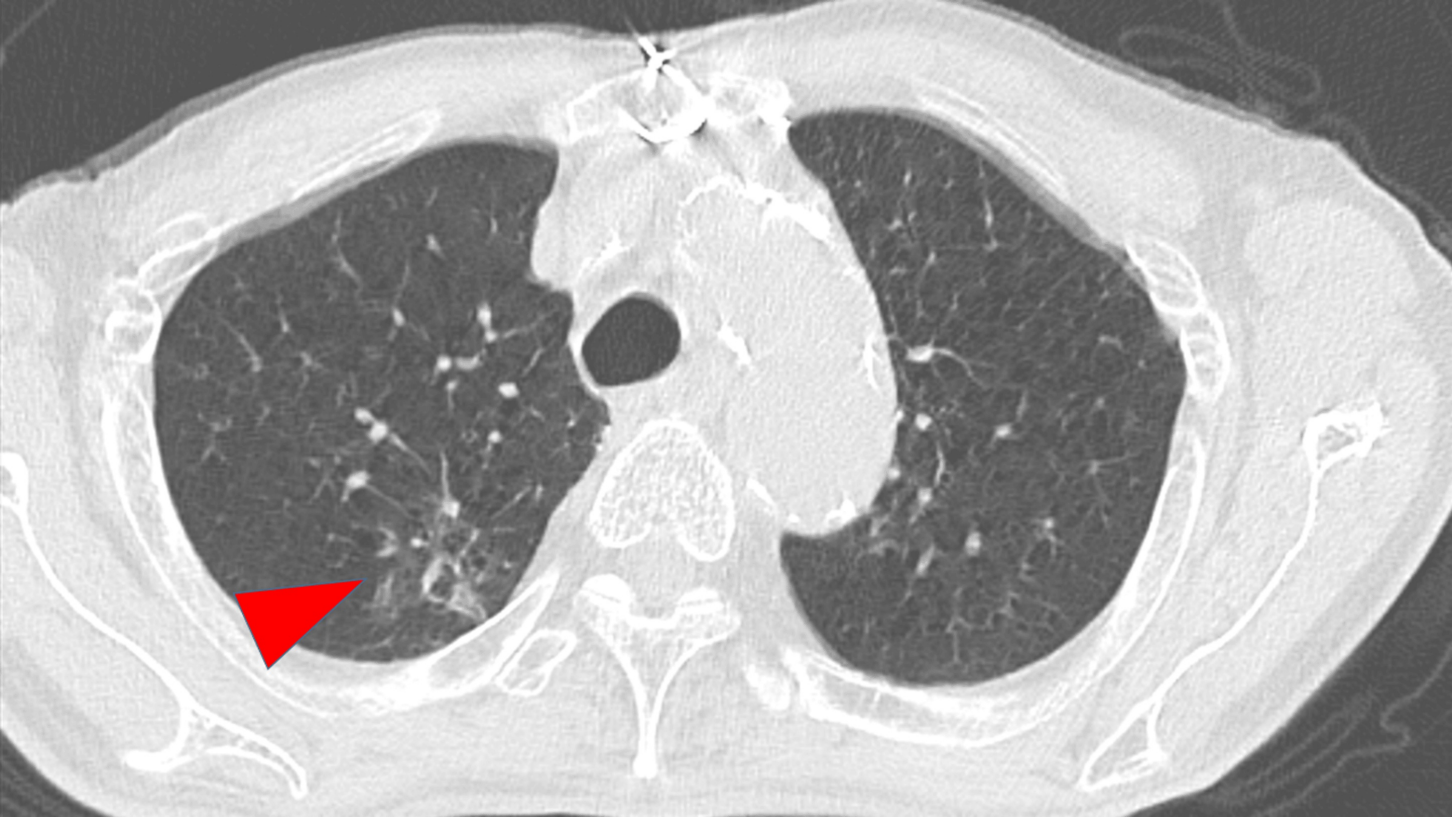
A lesion suggestive of lung cancer was observed in the right upper lobe on chest CT.
